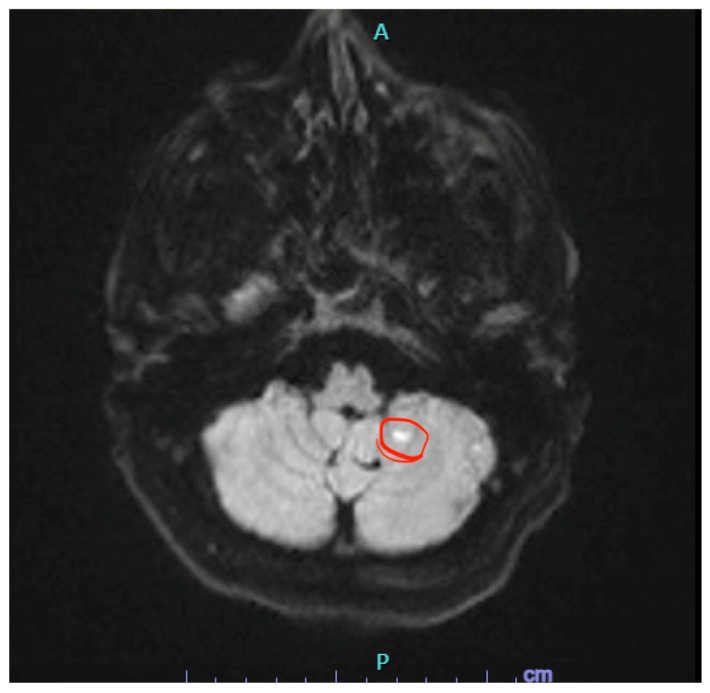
Diffusion-weighted imaging magnetic resonance image demonstrating the small stroke of the left cerebellum.
